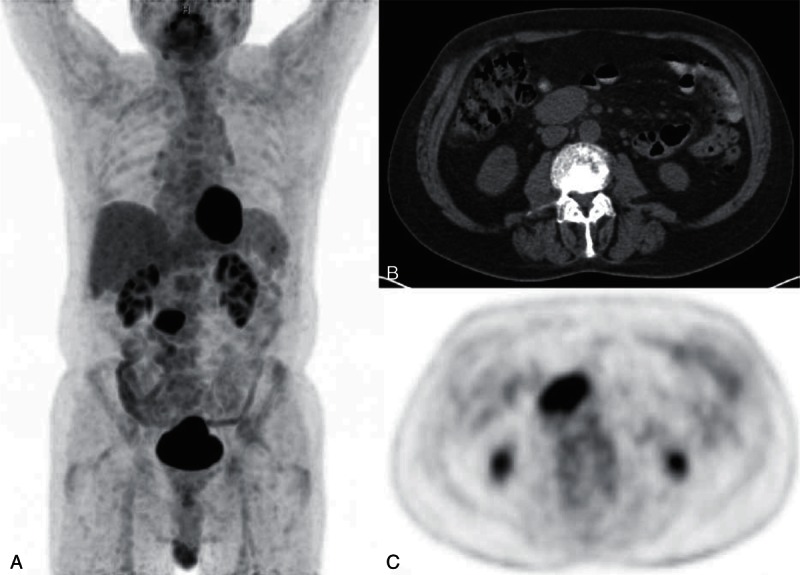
F-18 FDG PET/CT maximum intensity projection (A), CT for attenuation correction (B), and PET (C) images show the mass was intensely FDG avid (SUVmax 6.6). No enlarged or FDG avid lymph node was present elsewhere above or below the diaphragm. F-18 FDG PET/CT = fluorine-18-labeled fluorodeoxyglucose positron emission tomography with computed tomography for attenuation correction.
